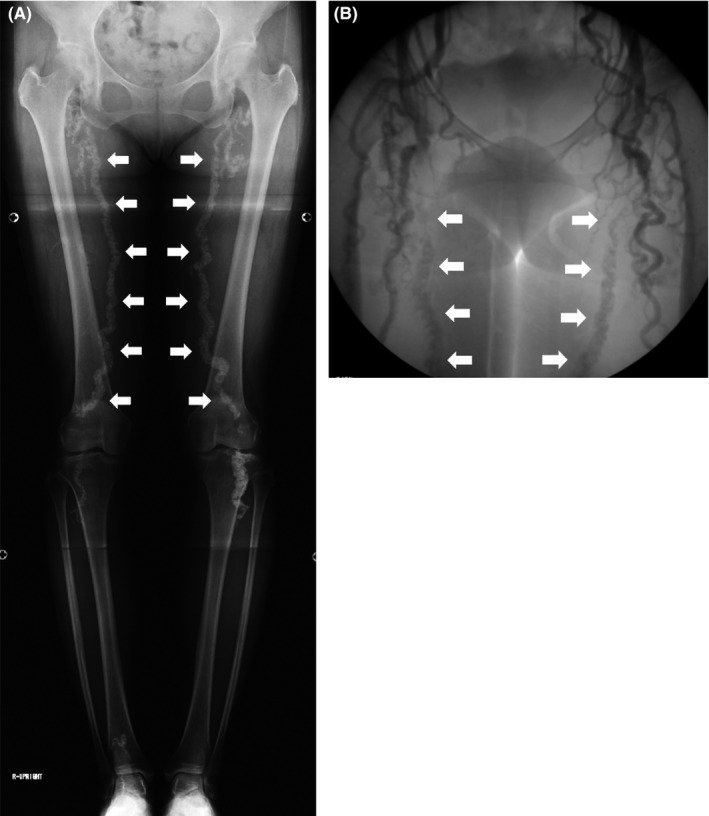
A, Plain radiographs of the lower limbs showing “rail‐tracking calcification” (arrows) of the femoropopliteal arteries bilaterally. B, Peripheral angiogram showing total occlusion of bilateral femoral arteries (arrows) and development of numerous collateral arteries
